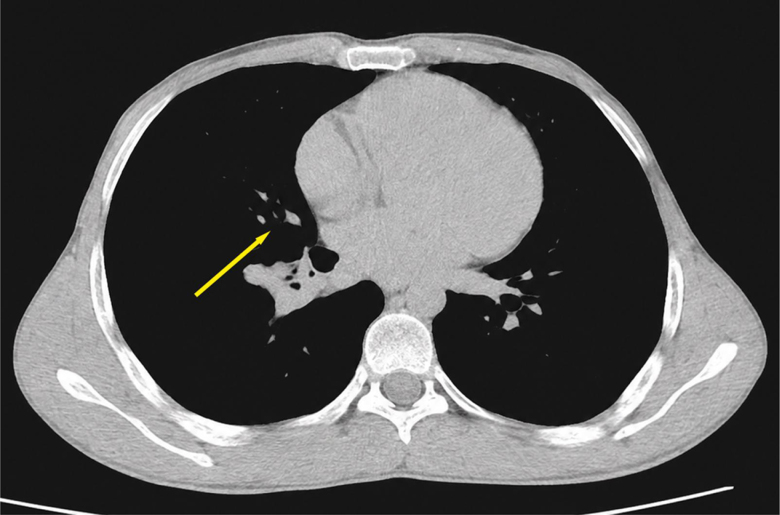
Contrast-enhanced chest CT scan. The images display filling defects within the branches of the right pulmonary artery. CT = computed tomography
